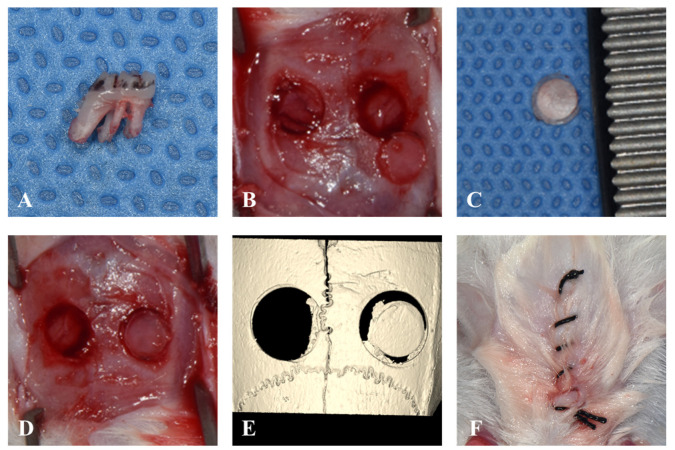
Surgical procedure. (A). Extraction of maxillary first molar without any root fractures. (B). Two defects in the calvaria were created by A 4 mm trephine burr. (C). Harvested autogenous bone from the calvaria. (D). The autogenous bone was grafted in the right calvaria defect. (E). μ-CT images taken immediately after the surgery. (F). The suture line located on the mid-sagittal area to avoid the surgical area.
